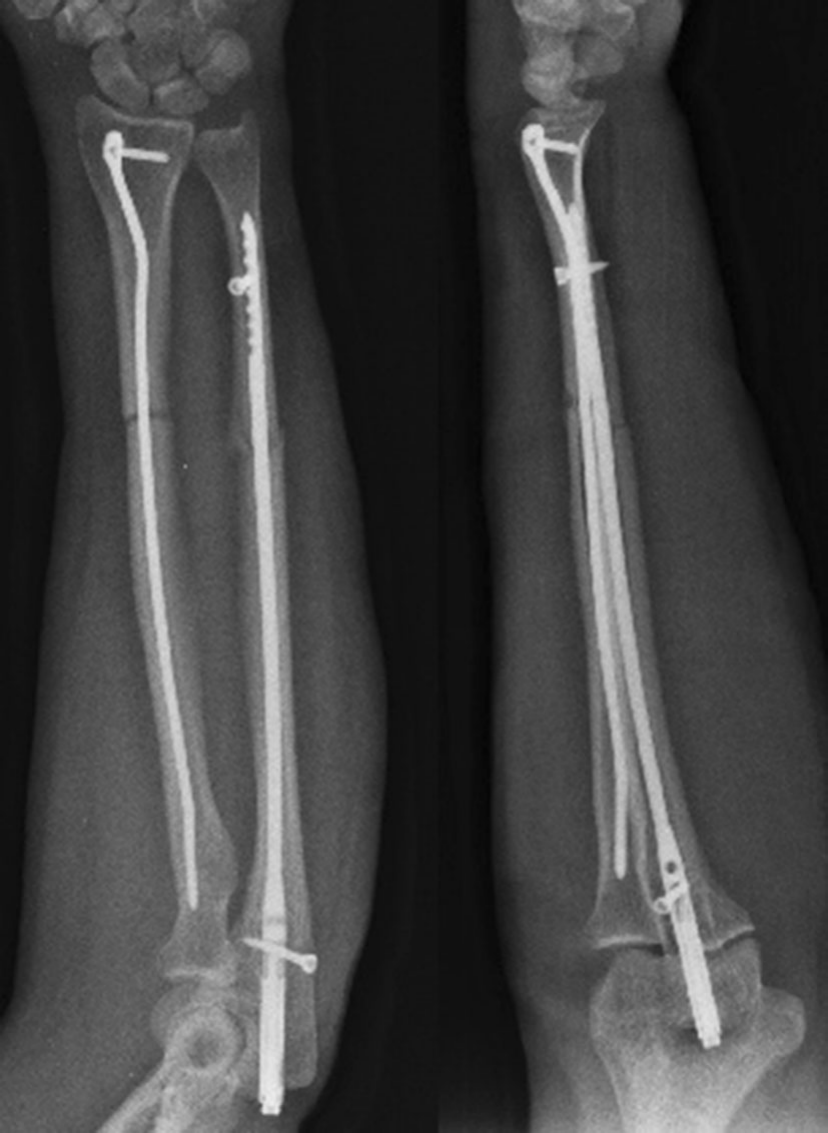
Radial inclination is maintained and compression applied to the ulna fracture line from the proximal can be seen in patient’s postoperative AP and lateral radiograph
